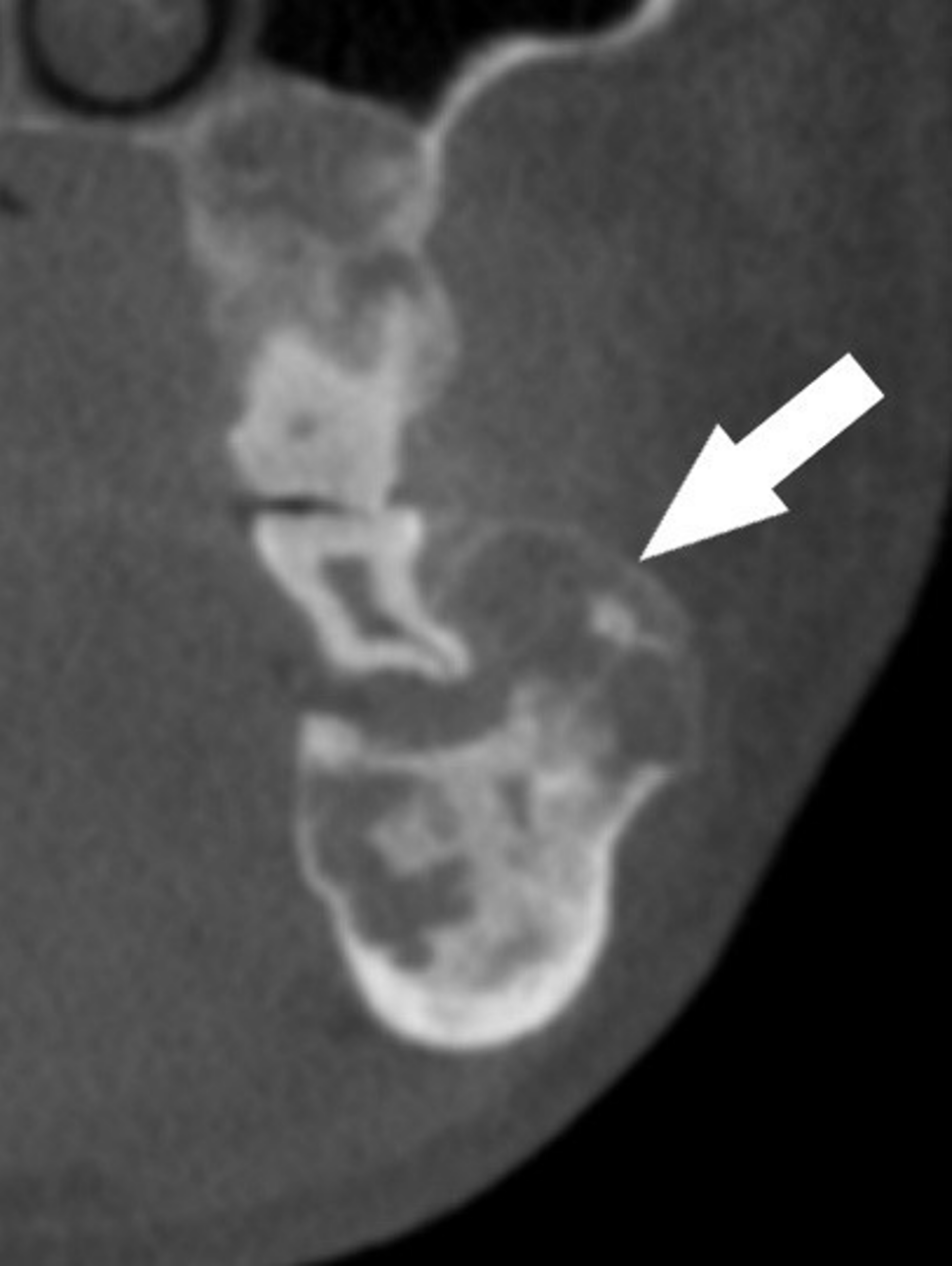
Coronally reconstructed CBCT of the left side of the mandible: depicting thinning of the buccal cortical plate. CBCT, cone beam CT.
